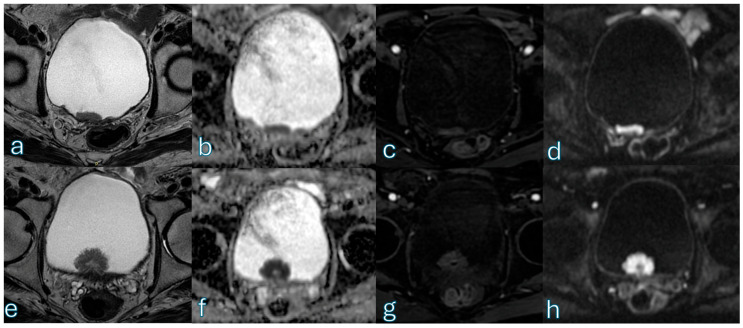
Multiparametric MRI in a 70-year-old patient demonstrates two synchronous bladder lesions with distinct morphologic and functional characteristics. The first lesion (a–d) is a flat bladder wall lesion, measuring approximately 1 cm, with equivocal findings on T2-weighted imaging (T2WI-(a)) and DWI/ADC (b,c). In this case, dynamic contrast-enhanced (d) imaging demonstrates early enhancement extending toward the muscular layer, which was fundamental for lesion characterization and supports classification as VI-RADS 3. The second lesion (e–h) is a pedunculated mass arising from the bladder wall, measuring approximately 2 cm, showing the characteristic inchworm sign on diffusion-weighted imaging (f) with corresponding findings on the ADC map (g), orienting the assessment toward a VI-RADS 2 category despite its size.
